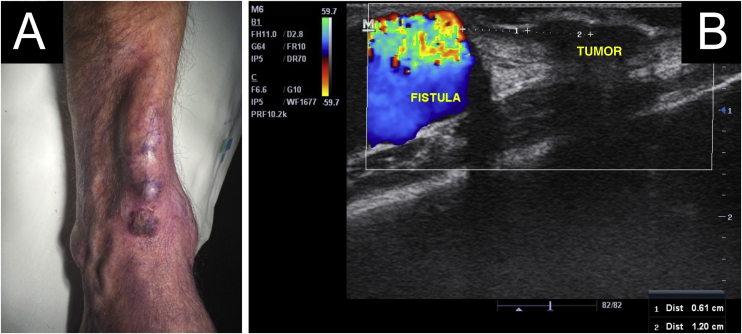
(A) Tumor located distally to an active AVF. (B) Dermatological ultrasound (Mode B and Mindray Doppler, 16 MHz linear probe). Tumor 0.6 cm away from the active fistula.
